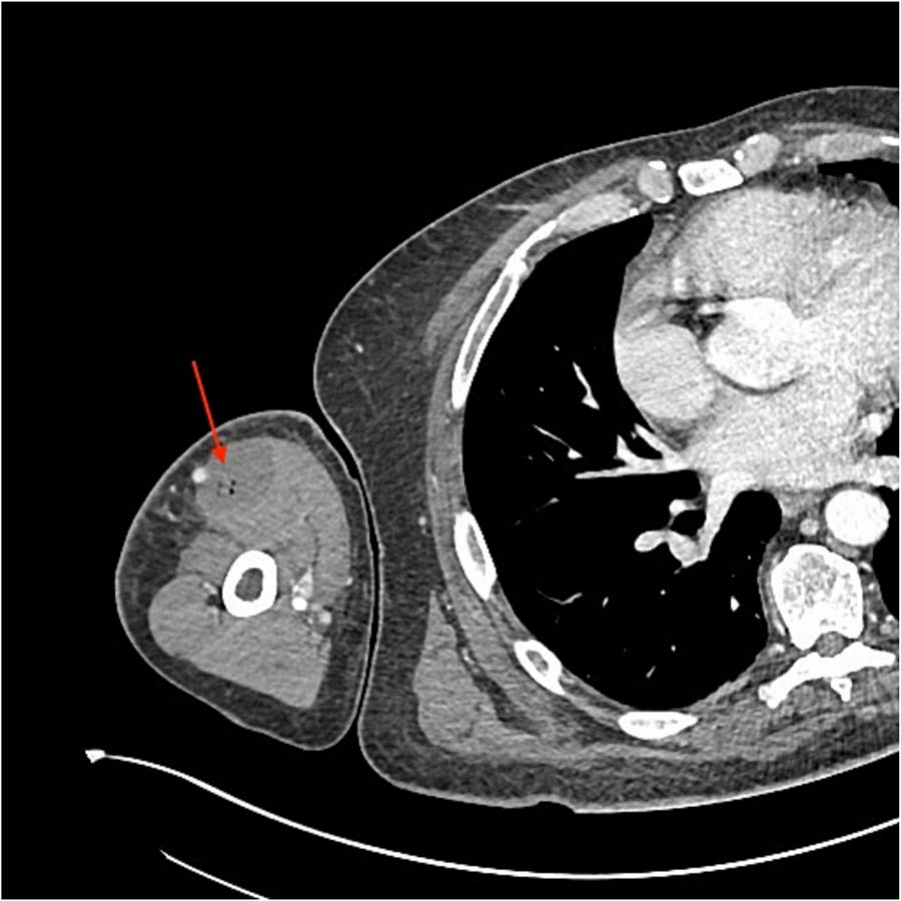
CT scan showing gas loculi within the biceps (arrow).
